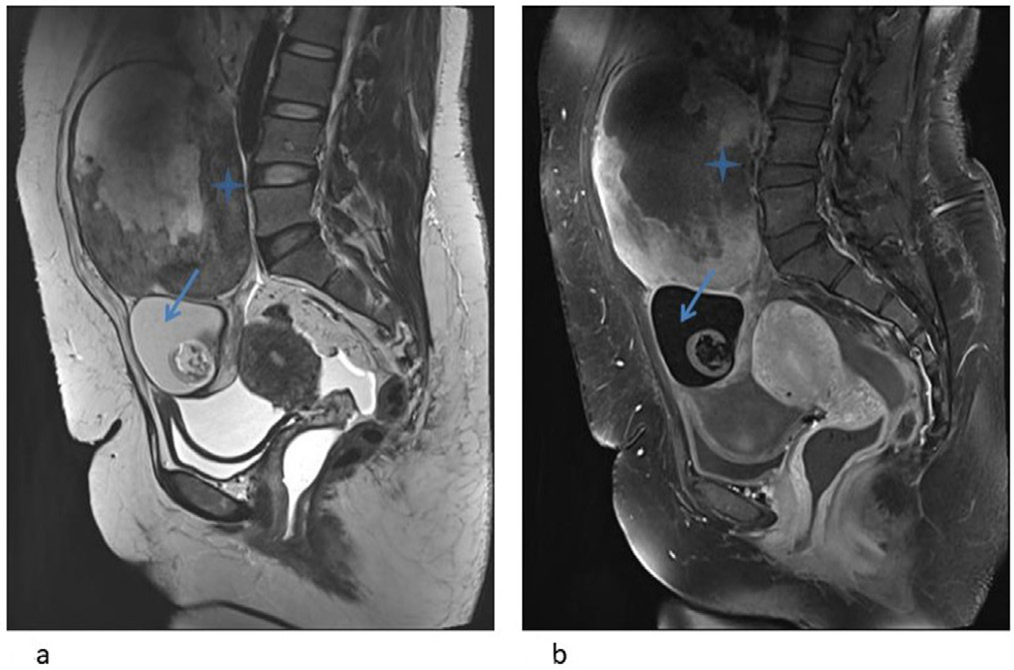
(a, b): Sag MRI - T2 and T1FS post-contrast images showing
